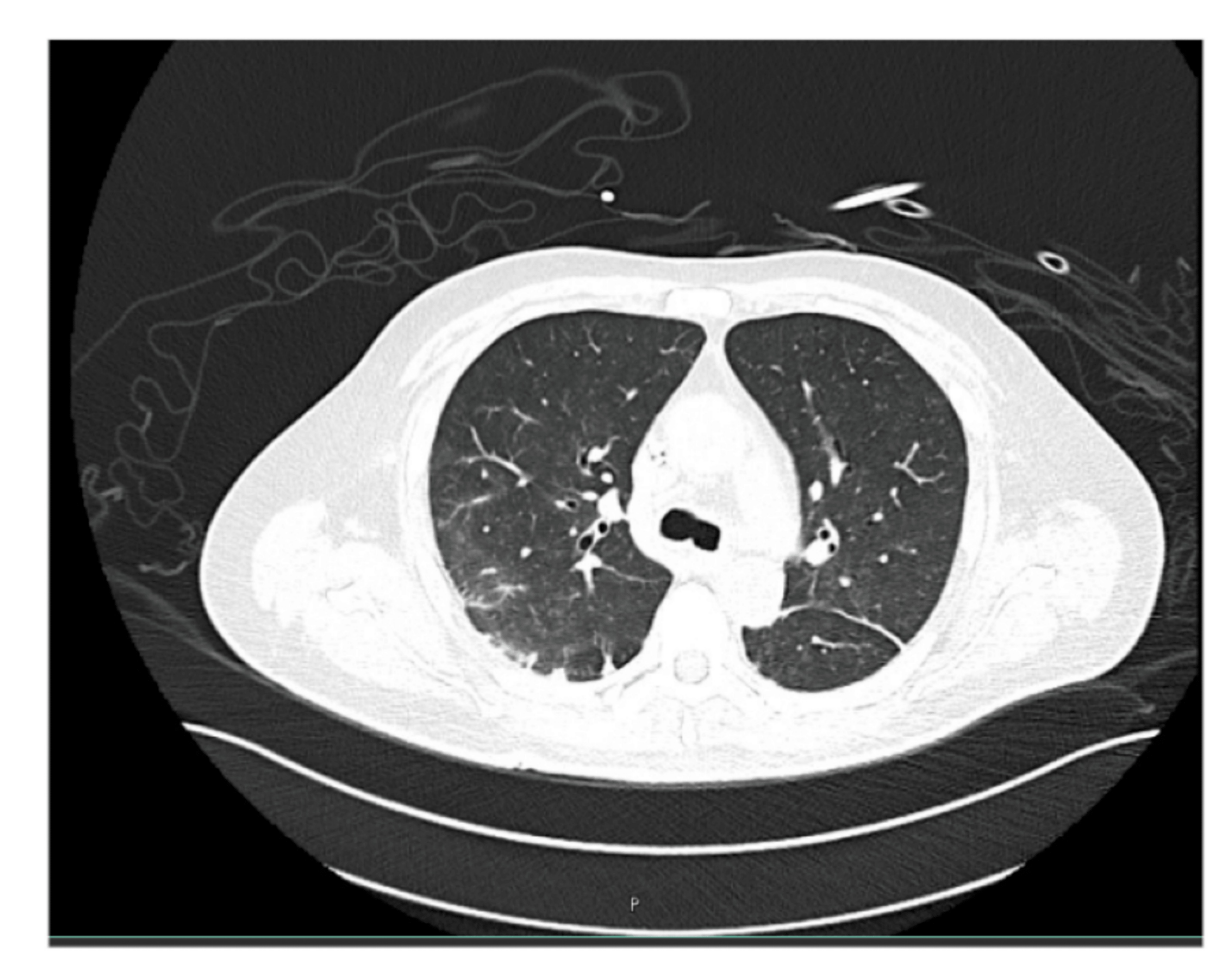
Axial CT scan of the upper lobes. Micronodules in a centrilobular distribution with a tree-in-bud pattern, predominantly in the right lung, along with dilated bronchi in the upper lobes.
